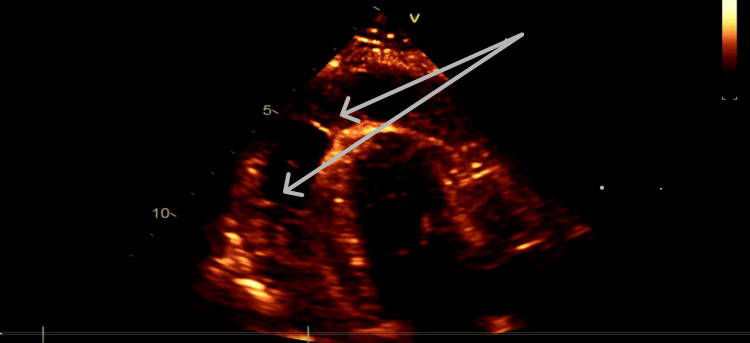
Echocardiography demonstrating pericardial effusion compressing the heart with fibrinous strands Global pericardial effusion with fibrinous strands compressing the myocardium
